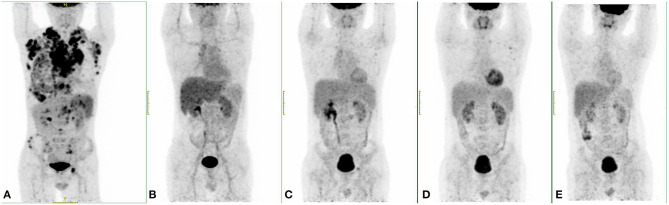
PET/CT results of this case at different time points. (A) The PET/CT image when diagnosed. (B) A PET/CT examination after the fourth cycle of ABVD (doxorubicin, bleomycin, vinblastine, dacarbazine) showed complete remission (CR). (C) After programmed death-ligand 1 (PD-L1) monoclonal antibody (mAb) treatment, there were still multiple swollen lymph nodes in the PET/CT results, and new lesions were found in the pelvic cavity behind the peritoneum. (D) After two doses of programmed death-1 (PD-1) mAb treatment, PET/CT examination showed that the size of the retroperitoneal and pelvic lymph nodes was smaller, but new lesions occurred in the left clavicular lymph nodes. (E) After autologous stem cell transplantation (ASCT), this patient reached CR.
